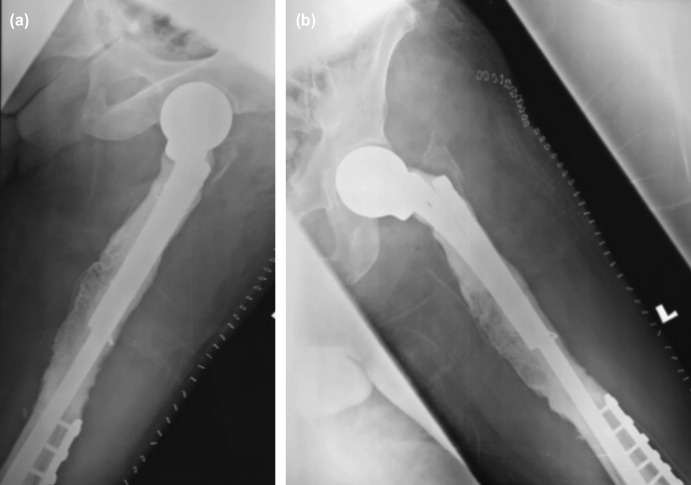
Post-operative radiographs (a) and (b) showing the modified unipolar hemiarthroplasty (MUH) construct. A 9-hole distal femur buttress plate with cement augmentation used to address the lytic lesions which involved the shaft and distal femur.
